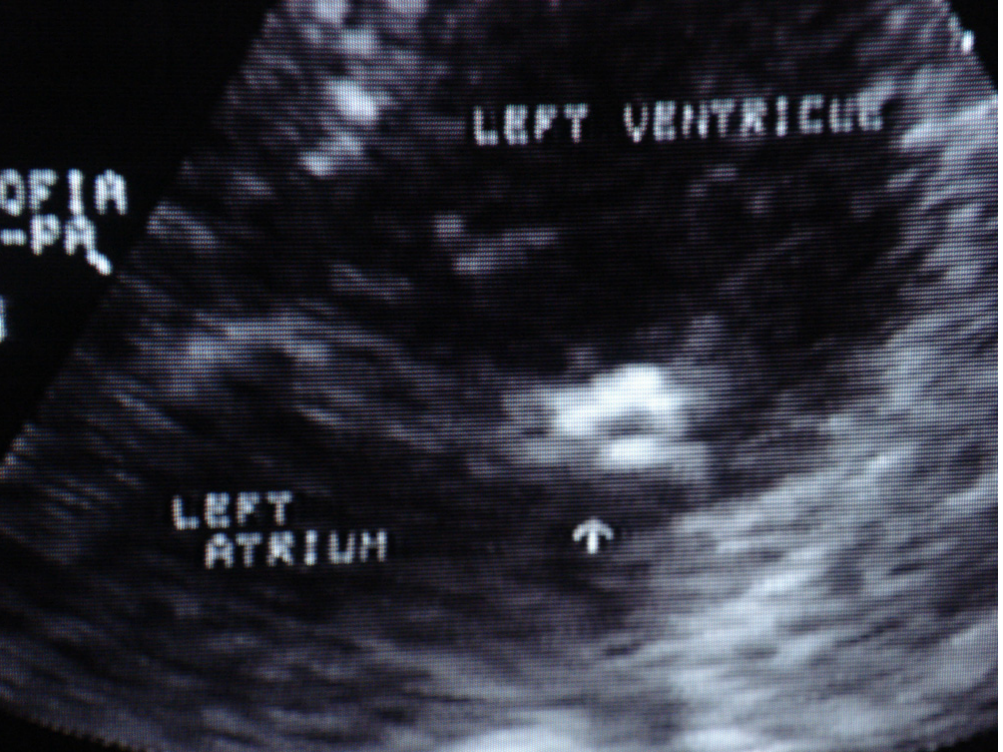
apical four chamber view.
